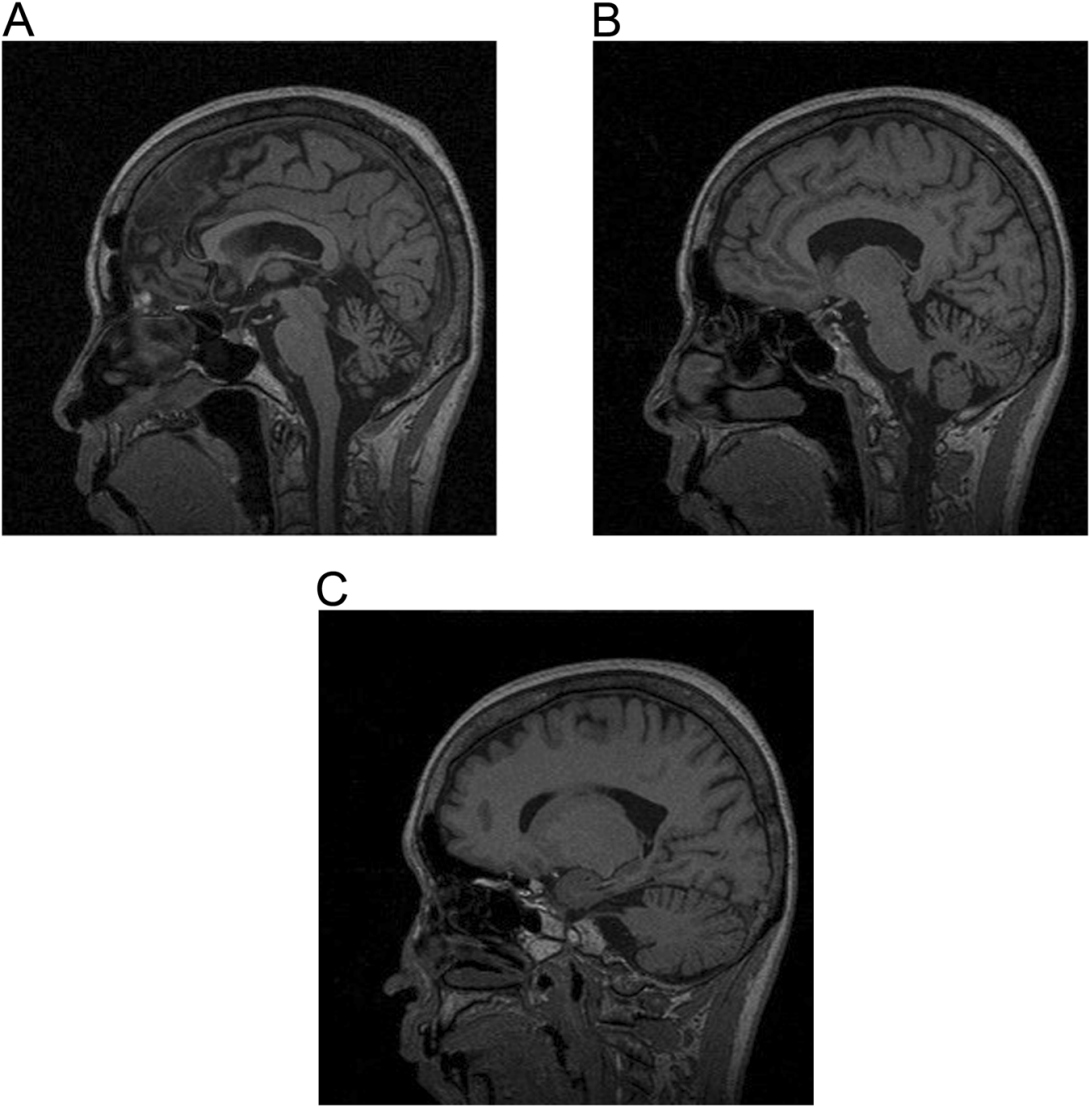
T1-weighted magnetic resonance imaging of an SCA31 patient (female, 65 years old) sectioned in the sagittal pane (A: near midline, B: paramedian, C: lateral sections). At a near midline plane, the culmen shows prominent atrophy and the declive, folium, tuber vermis also appear atrophic (A). At the lateral planes (B, C), the cerebellar atrophy is obvious only the lobulus quadrangularis
